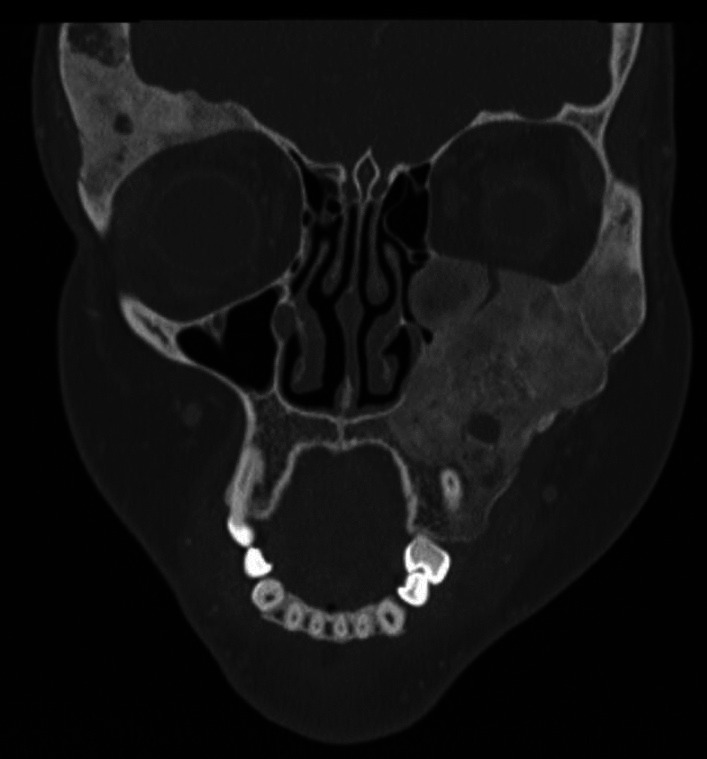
A coronal CT slice of paranasal sinuses of a 19-year-old female patient with polyostotic FD. Image shows lesions involving the left maxilla, mandibula, hard palate, zygomatic bone, temporal bone, left and right sphenoid bones, as well as the right frontal bone and the occipital bone. Lesions exhibit a typical ground-glass appearance and obliterate the left maxillary sinus, elevate the floor of the orbit and extend into the alveolar bone. During follow-up the lesions were found to grow rapidly, causing pain and swelling
